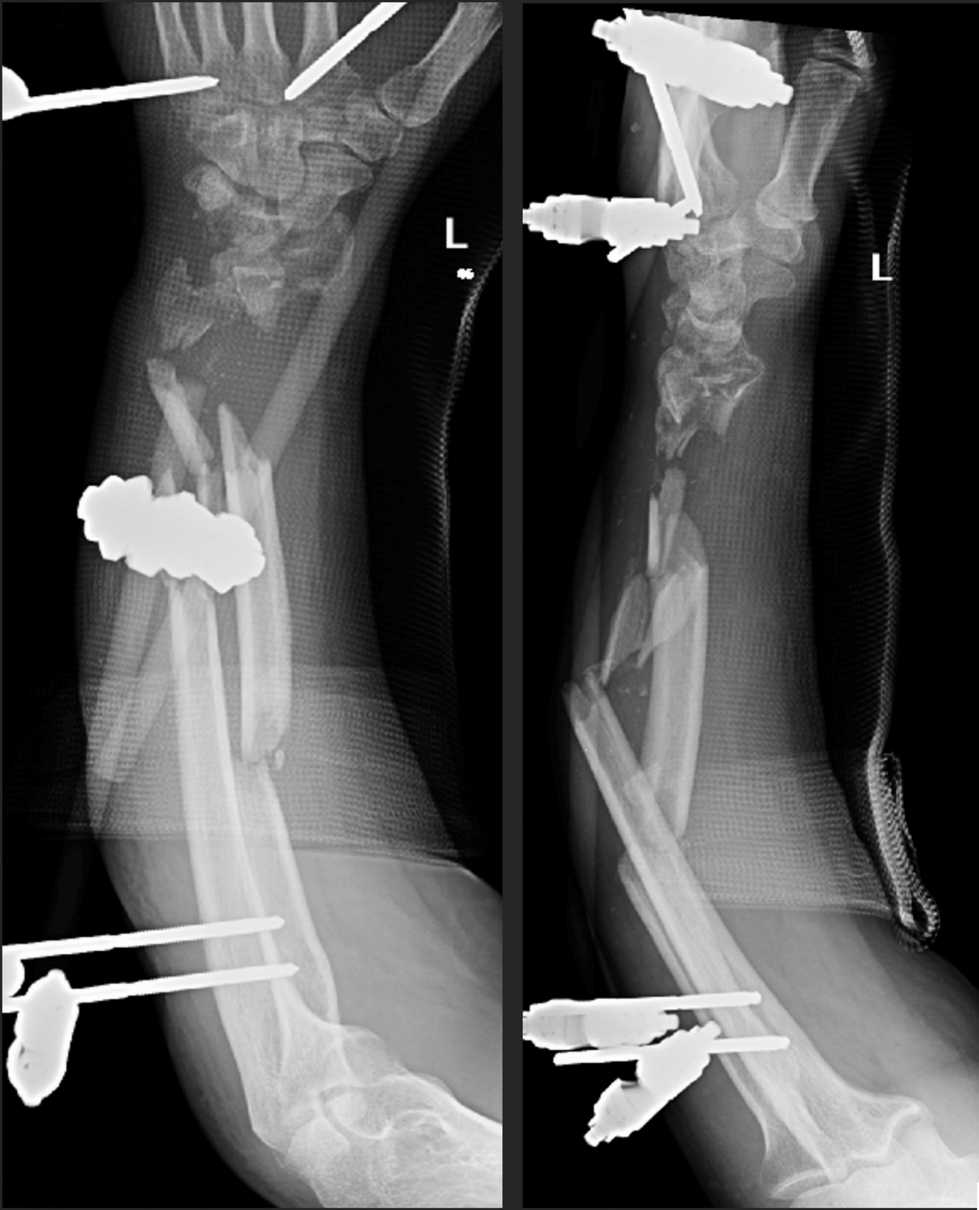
Anteroposterior (AP) and lateral radiographic views of the left forearm following Kirschner wire (K-wire) removal and application of external fixation.
